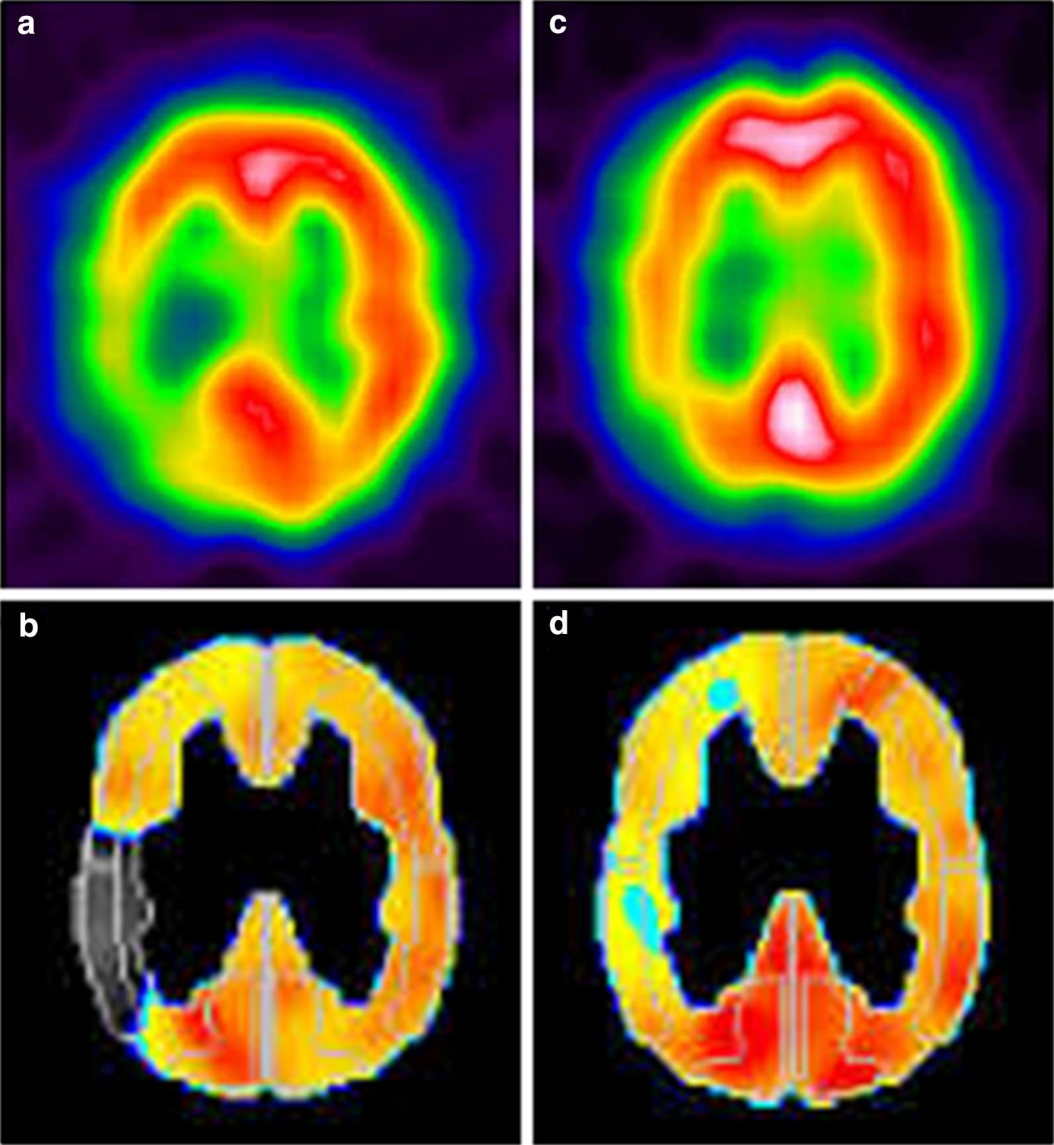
Preoperative SPECT revealed the localized hypoperfusion in the right posterior MCA territory (a), and decreased CVR was detected by ACZ challenge (b). Improvement of the CBF (c) and CVR (d) was identified on postoperative SPECT
